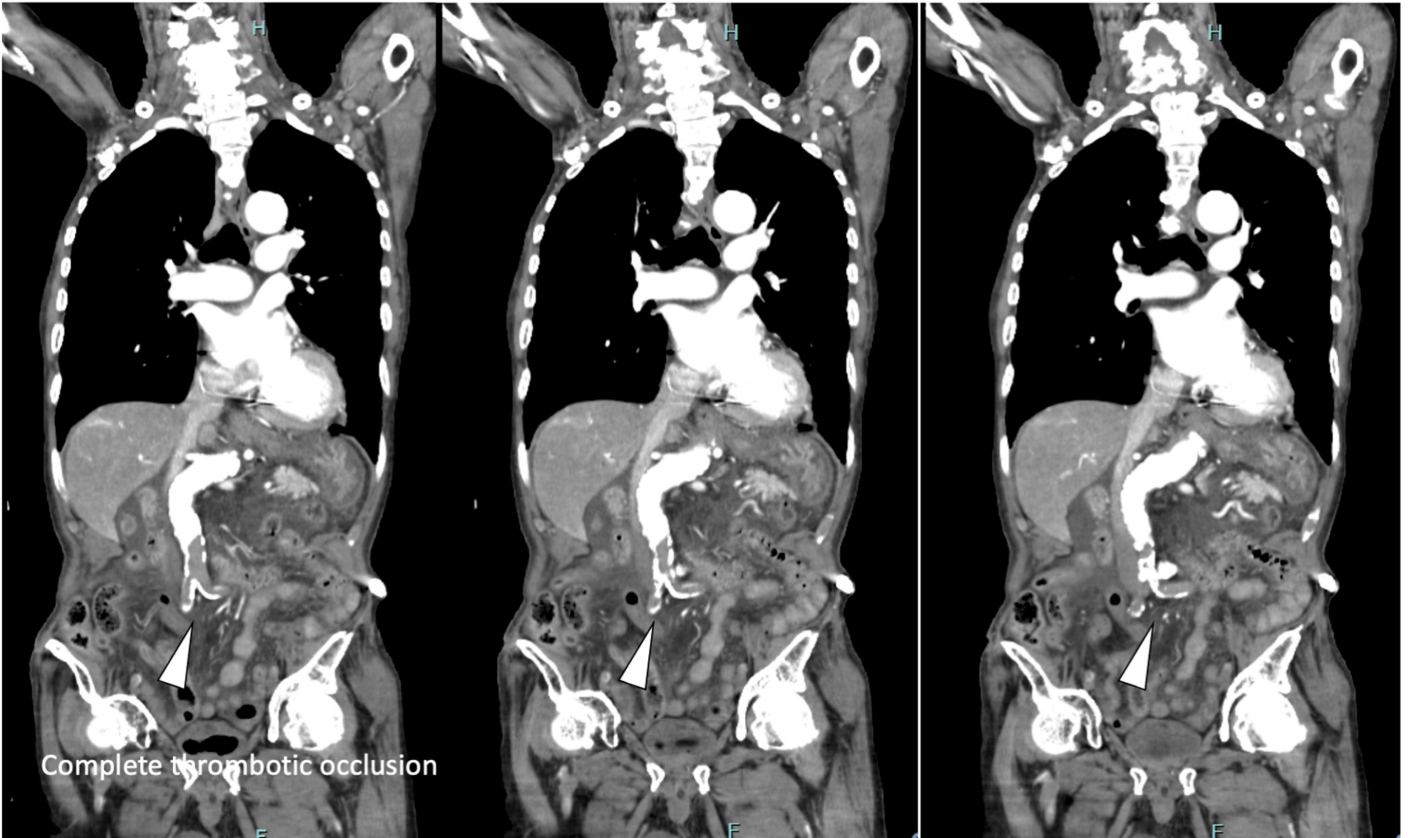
Arterial phase coronal CT images showing complete abdominal aortic occlusion extending to the common iliac arteries. Serial contrast-enhanced coronal CT images of the abdomen in the arterial phase on day four of hospitalization showing complete thrombotic occlusion of the abdominal aorta extending to the bilateral common iliac arteries (arrowheads). No contrast enhancement is observed distal to the level of occlusion, indicating the absence of arterial blood flow. These findings in the arterial phase are consistent with acute thrombotic occlusion of the abdominal aorta and explain the patient's abrupt bilateral lower limb paralysis and absent femoral pulses.
